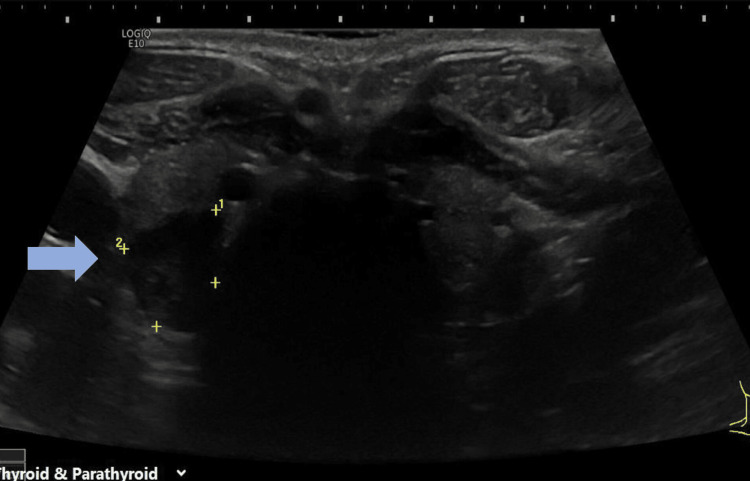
Ultrasound shows a parathyroid adenoma (17.5 mm × 10.5 mm × 8.5 mm) that lay adjacent to the lower pole of the right thyroid lobe.
